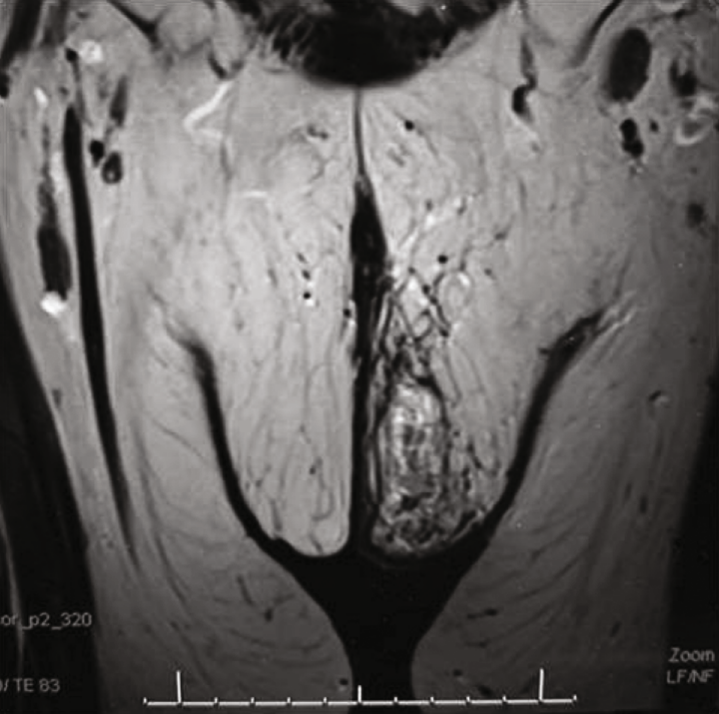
MRI showing an empty cavity without any collection. Skin infiltration with suspicion of a cellulitis. Absence of fistula.
